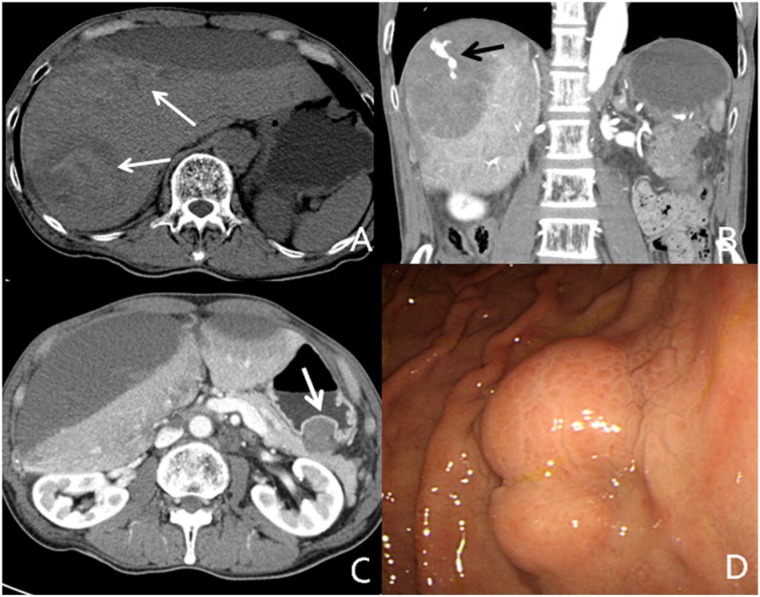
A 65-year-old man with a surgically diagnosed rupture of a hepatic metastasis from a gastric GIST. (A) An axial unenhanced CT section shows a large subcapsular hematoma with high-density sentinel clots (arrow) in the right posterior hepatic lobe. (B) Contrast-enhanced CT with coronal reconstruction shows a spherical heterogeneous low-density hepatic mass with active contrast leakage (arrow) adjacent to the hematoma, which was diagnosed as a ruptured hepatic metastasis after resection. (C) An abnormal low-density gastric mass is shown in the posterior body with normal overlying gastric mucosa (arrow). (D) A dumbbell-shaped submucosal mass is revealed by gastrofiberscopy at the level of the gastric body.
